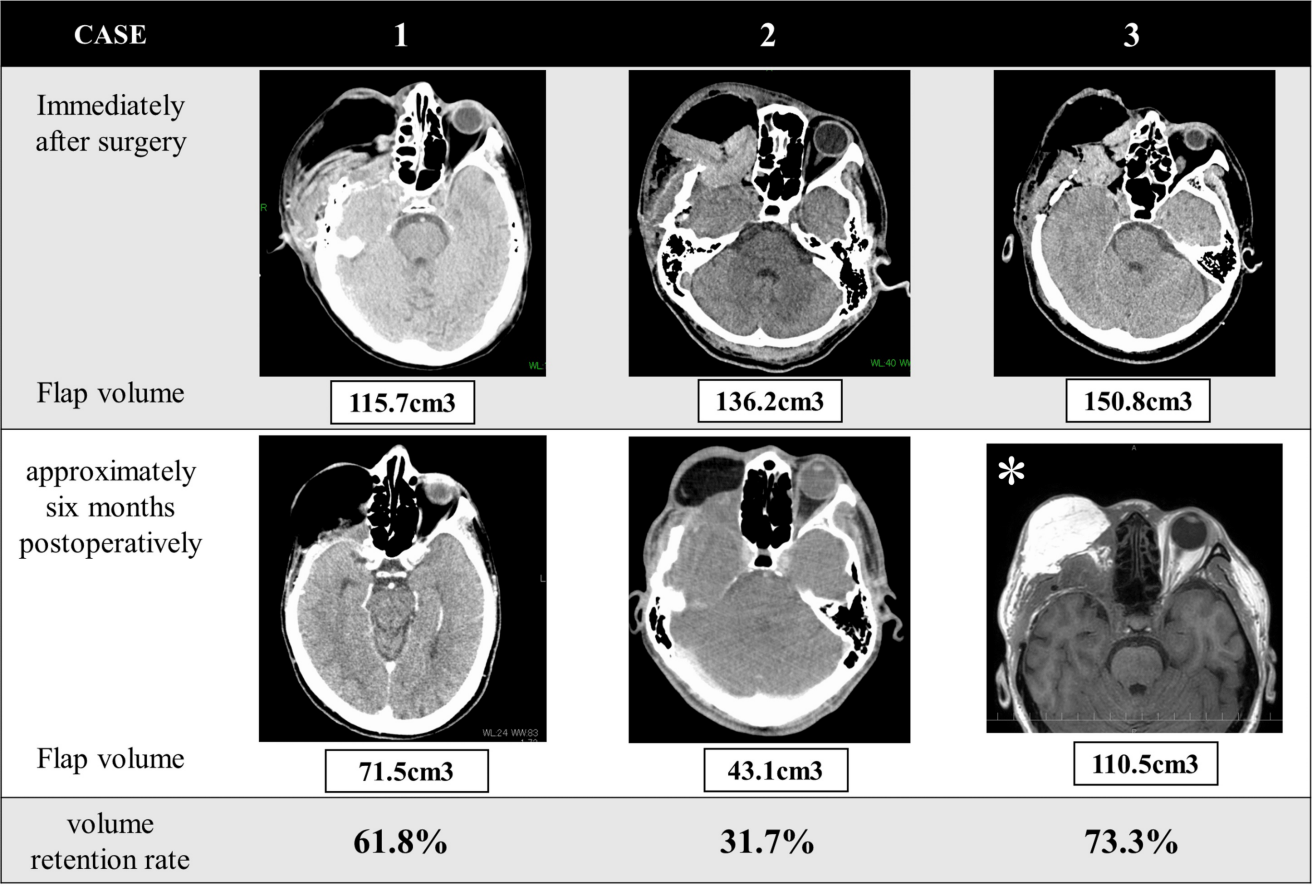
Volume change of the free flap The volumes of the transplanted rectus abdominis flaps are observed immediately after surgery and approximately 6 months postoperatively. The volume retention rates at approximately 6 months postoperatively were 61.8% for case 1, 31.7% for case 2, and 73.3% for case 3. Flap volumes were evaluated through image processing using the OsiriX Dicom Viewer (Pixmeo Inc., Genève, Switzerland). *Since CT imaging was not performed during the relevant period, the volume of the flap was measured using MRI images.
